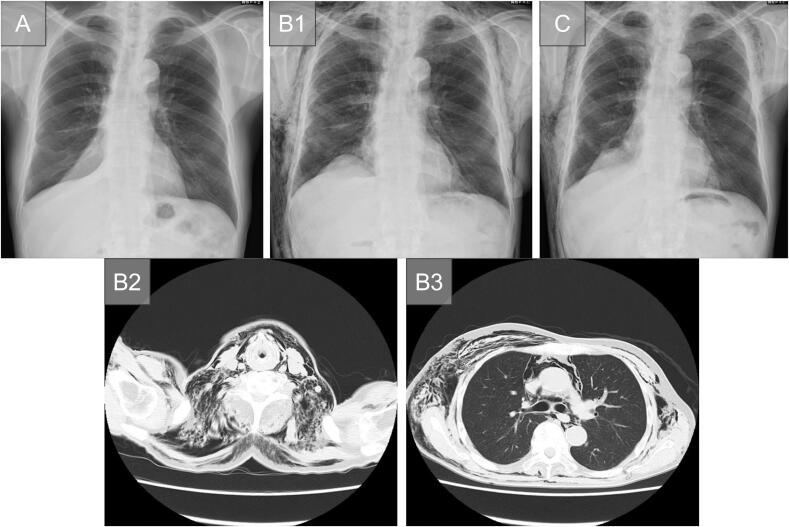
(Case 1) (A) Chest X-ray shows no subcutaneous emphysema prior to discharge (POD6). (B) (B1) Chest X-ray at readmission shows subcutaneous and mediastinal emphysema (POD7). Note the linear image of the pneumomediastinum along the left contour of the heart. Chest CT at admission shows subcutaneous and mediastinal emphysema from the neck (B2) to the chest (B3) (POD7). No obvious pneumothorax was observed. (C) Chest X-ray shows a reduction in subcutaneous emphysema compared to that observed prior to discharge (POD11). Abbreviations: CT: computed tomography; POD: postoperative day.
